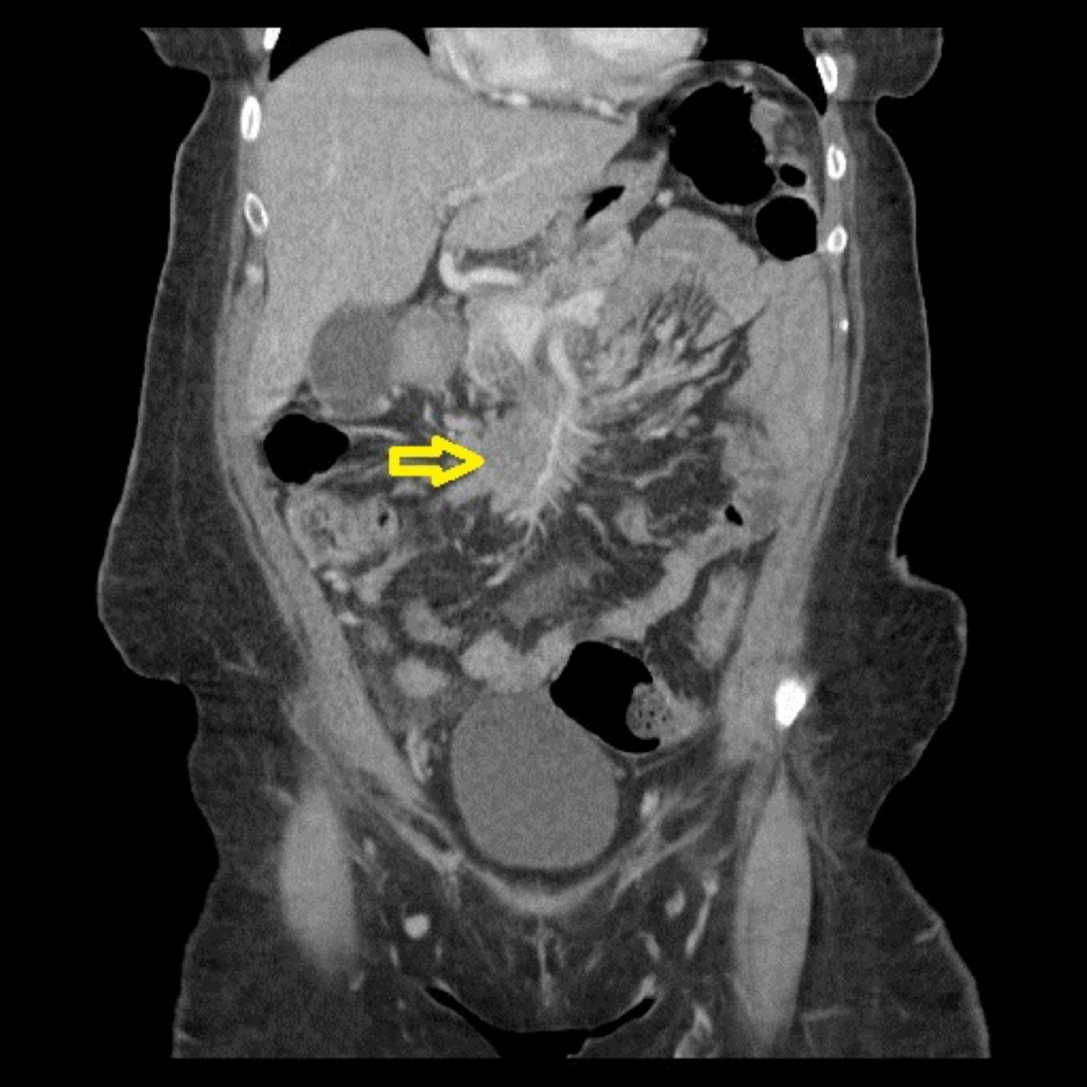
Initial CT of the abdomen and pelvis - axial view The image shows an eccentric mass and adenopathy encasing the superior mesenteric artery as depicted by the yellow arrow CT: computed tomography
